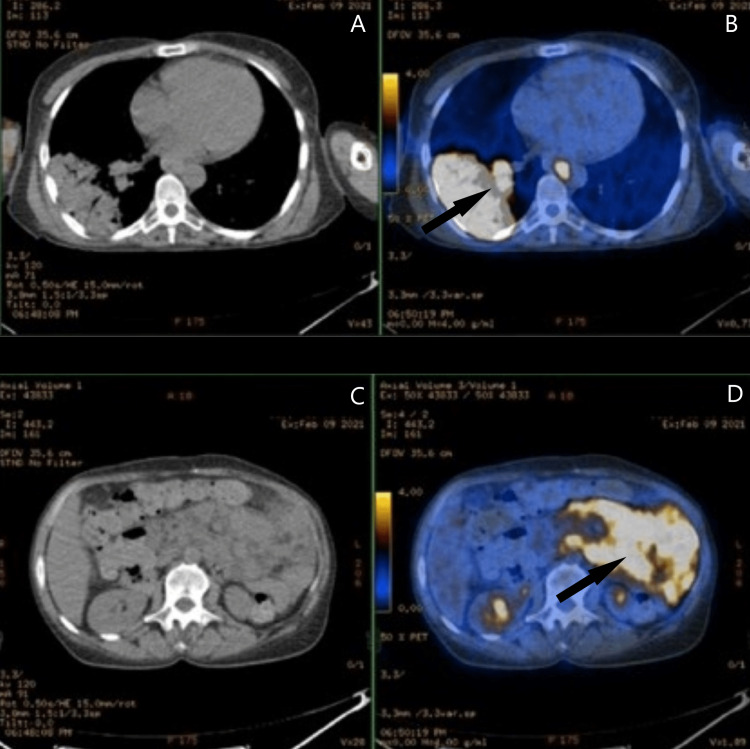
CT scan and FDG18-PET. A, B: Chest level showing right lower lobe lymphomatous infiltration (arrow). C, D: Abdominal level showing retroperitoneal adenopathy conglomerate (arrow). FDG18-PET = fludeoxyglucose F18-positron emission tomography
